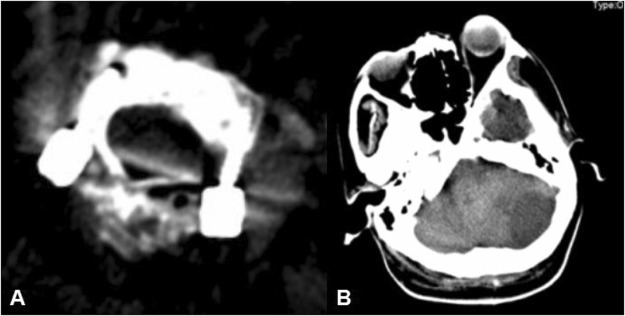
A 55 year-old female with atlantoaxial dislocation received free-hand C1 pedicle-C2 pedicle internal fixation. The bilateral vertebral arteries were involved, and the patient developed paralysis of the right upper and lower limbs approximately 4 hours postoperatively. A cerebral infarction was found in the computed tomography scan.
